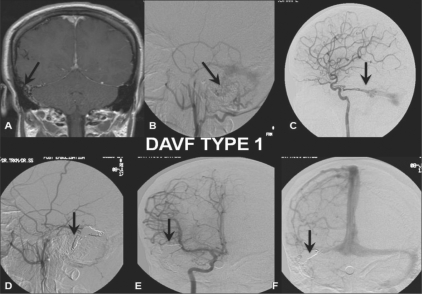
DAVF Type 1: Postcontrast coronal T1W MRI image (A) shows multiple tortuous flow voids (arrow) adjacent to the right sigmoid sinus. Selective right external carotid artery (ECA) (B) and internal carotid artery (ICA) (lateral view) (C) angiograms shows a DAVF type 1 with feeders (arrow) from the posterior meningeal branch of the middle meningeal artery and dural branches (arrow) from the cavernous ICA draining antegradely through the sigmoid sinus. Posttreatment selective right ECA (lateral view) (D) and ICA (anteroposterior view; arterial (E) and venous (F) phases) angiograms show coils (arrows) packing the right distal transverse and sigmoid sinus with total obliteration of the fistula
